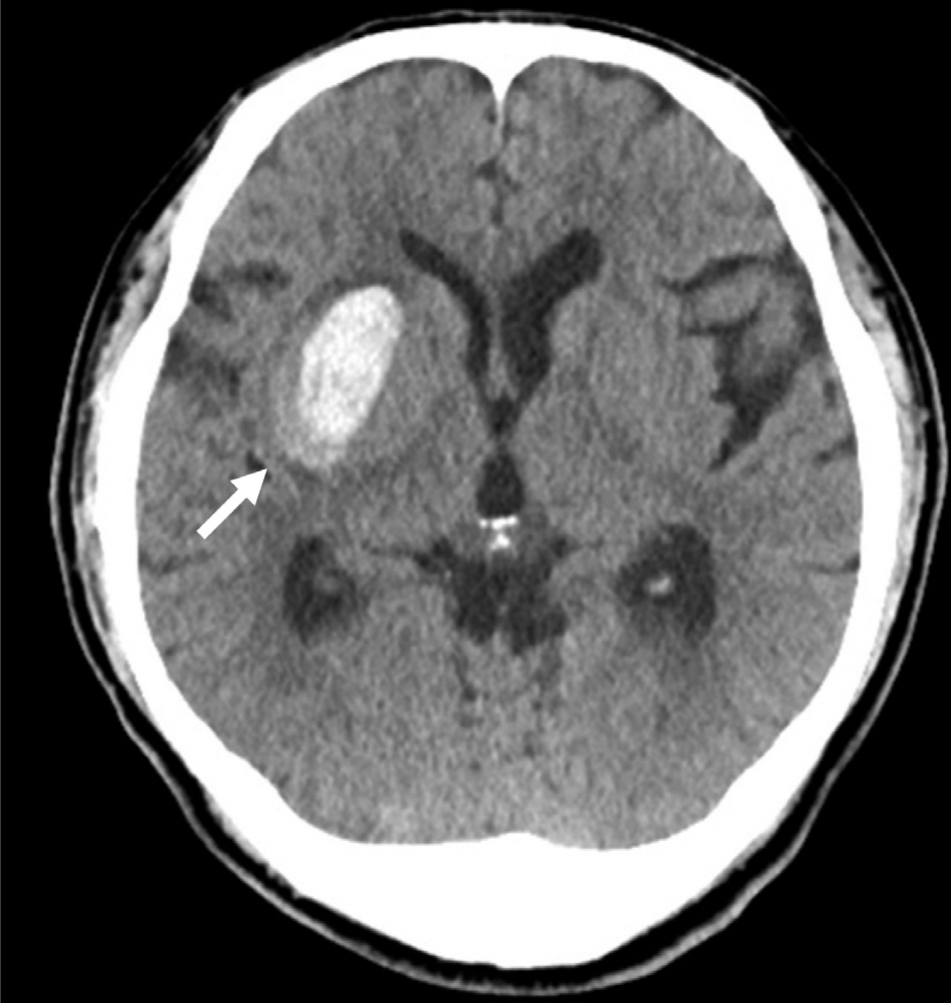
Computed tomography showing the right intracerebral hemorrhage (arrow).
